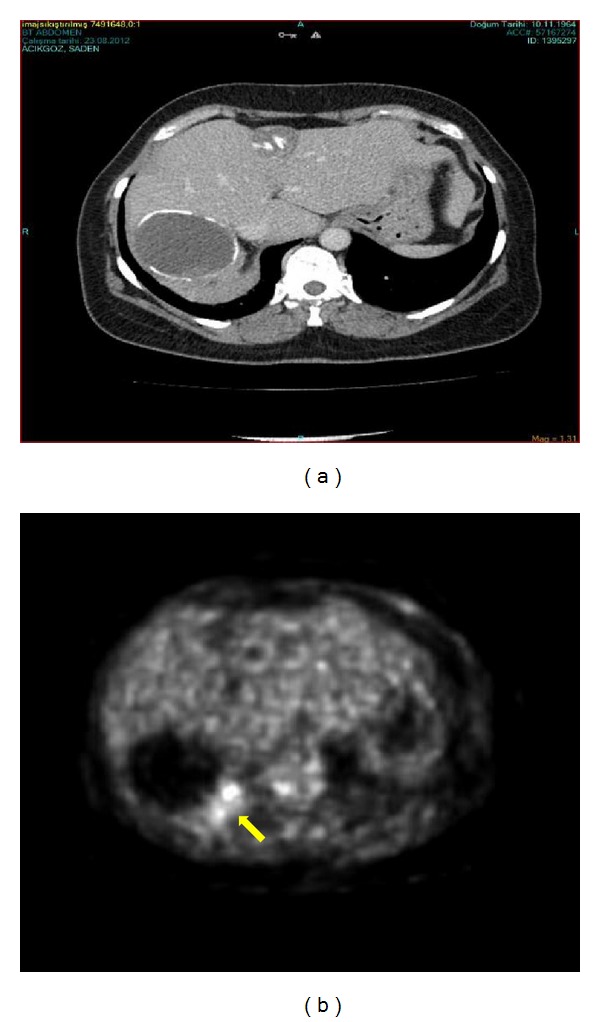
The radiological findings of the patient. (a) Abdominal computed tomography showing a 65 × 55 mm stage 5 hydatic cyst in the right hepatic lobe and a 30 × 28 mm in the left hepatic lobe, (b) FDG18 positron emission tomography of the patient on which the mass revealed a high activity of FDG. Yellow arrow shows the high suvmax activity area (suvmax 80).
